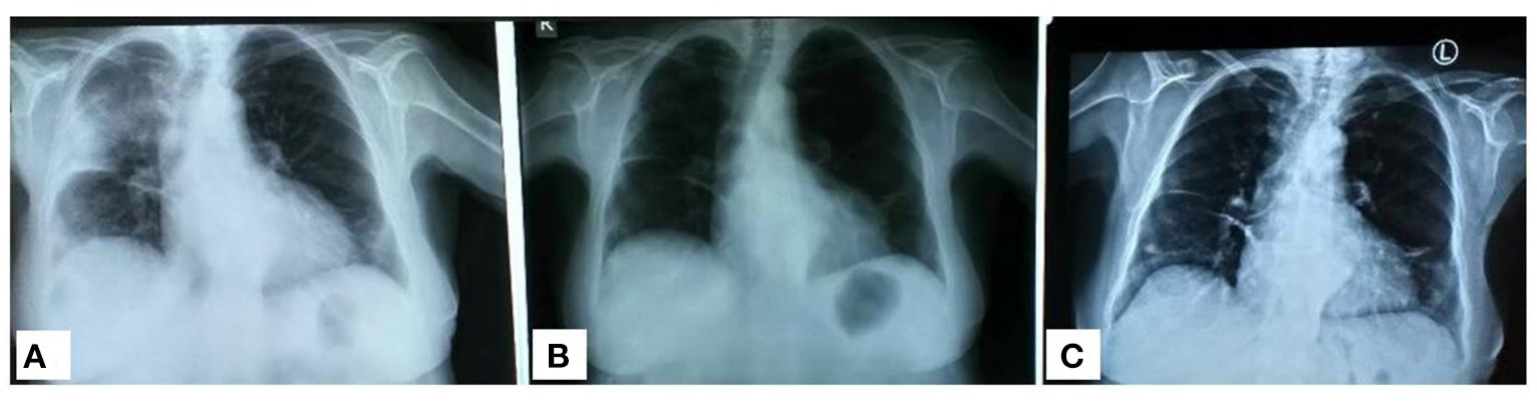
Serial thoracic X-rays that show the migration of the lesions. (A) Image on admission. (B) Picture after corticosteroid initiation as organizing pneumonia. (C) Picture 2 months after discharge.
